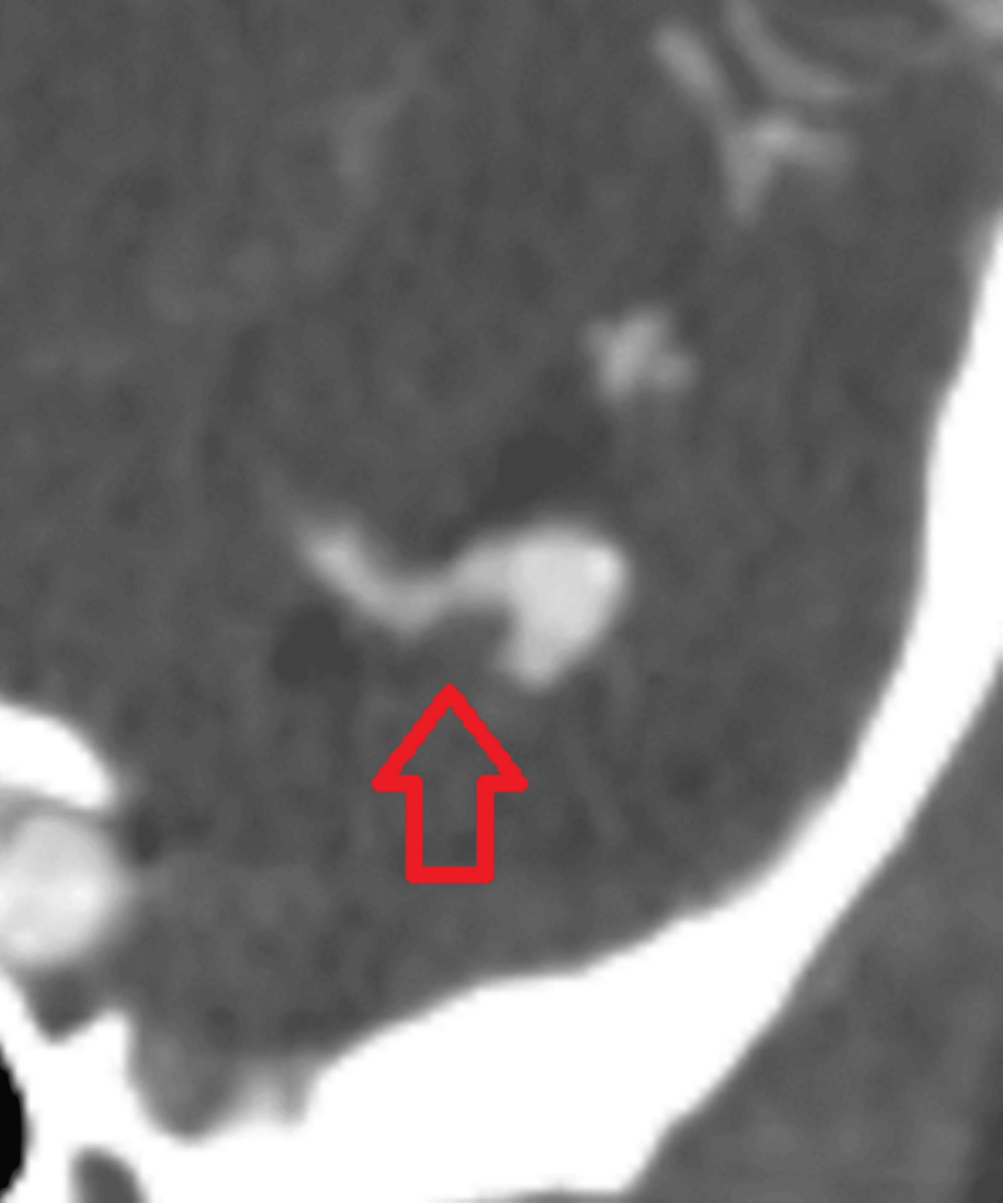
CTA demonstrating a possible filling defect in a saccular aneurysm previously thought to be a curvilinear partly fusiform-shaped aneurysm. Note the slight difference between the darker gray the arrow demarcates and the lighter gray above it. CTA: Computed tomography angiography
